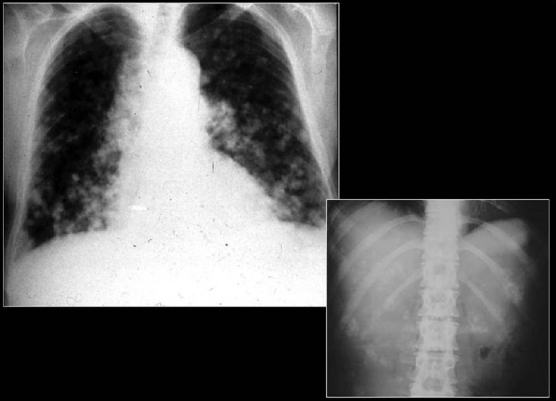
A PA chest radiograph of a patient with calcified metastases from a medullary carcinoma of the thyroid. A radiograph of the upper abdomen on the same patient showing calcified metastases to the liver
